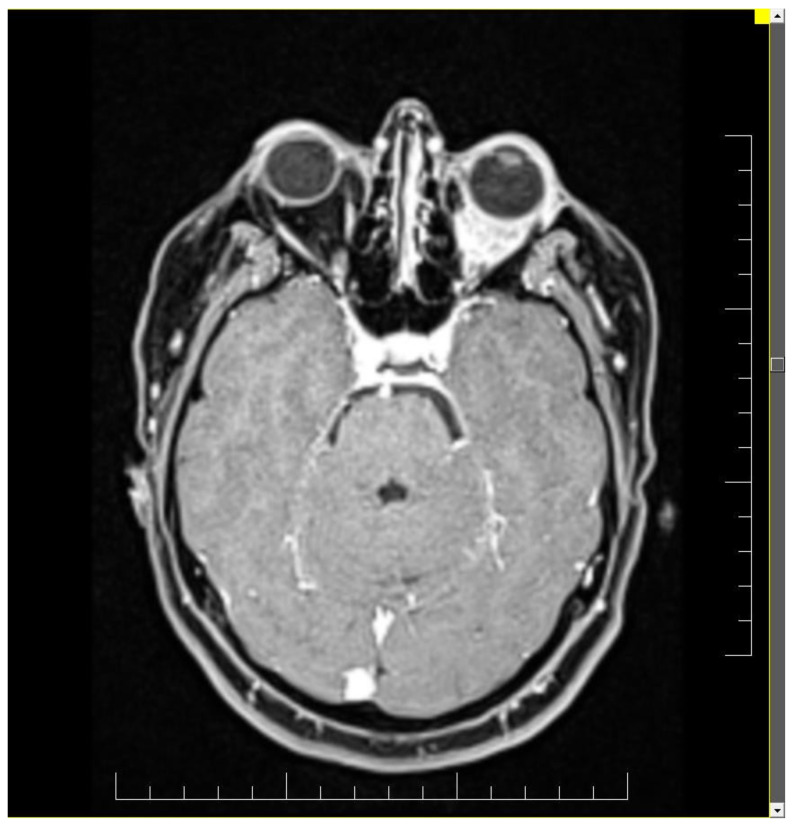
Brain CT scan showing an inhomogeneous mass with contrast agent capture in the retroocular space of the left orbit, cuffing the left optic nerve.
